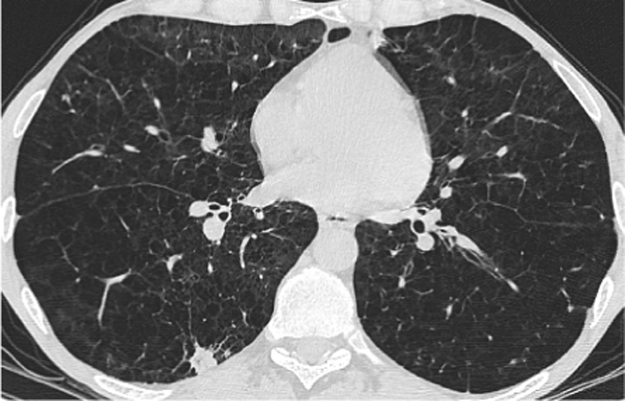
CT thorax from November 2016 showing resolution of previous inflammatory nodules.
